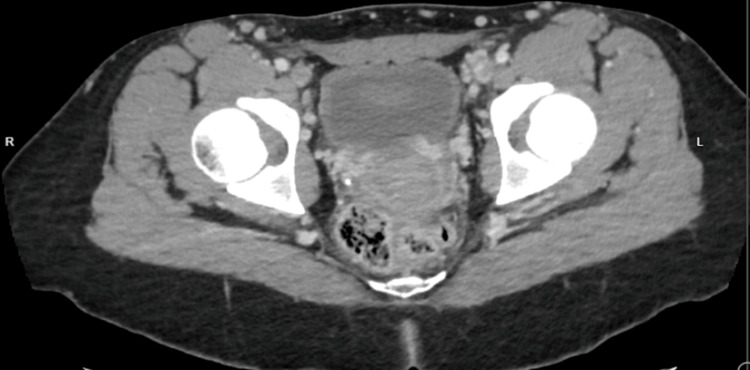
Follow-up axial computed tomography (CT) scan cut at the level of the pelvis demonstrates a filling defect at both external iliac veins bilaterally.
